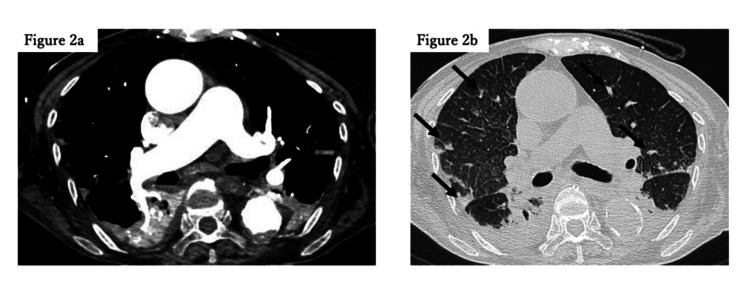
Contrast-enhanced axial CT 2a: Contrast-enhanced axial CT showed no massive thrombus occlusion in the pulmonary artery; 2b: Localized infiltrative shadows in the bilateral peripheral lung fields
